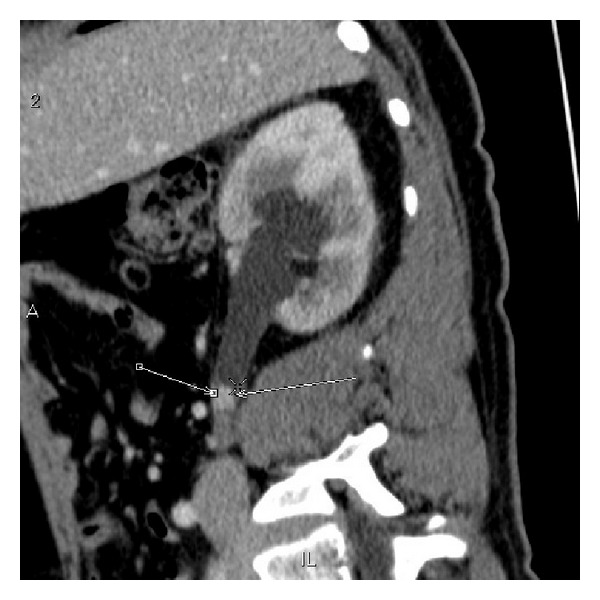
Portal venous phase CT (oblique sagittal MPR) showing an enhancing 9 mm soft tissue lesion (arrows) obstructing the lower moiety ureter right kidney.
